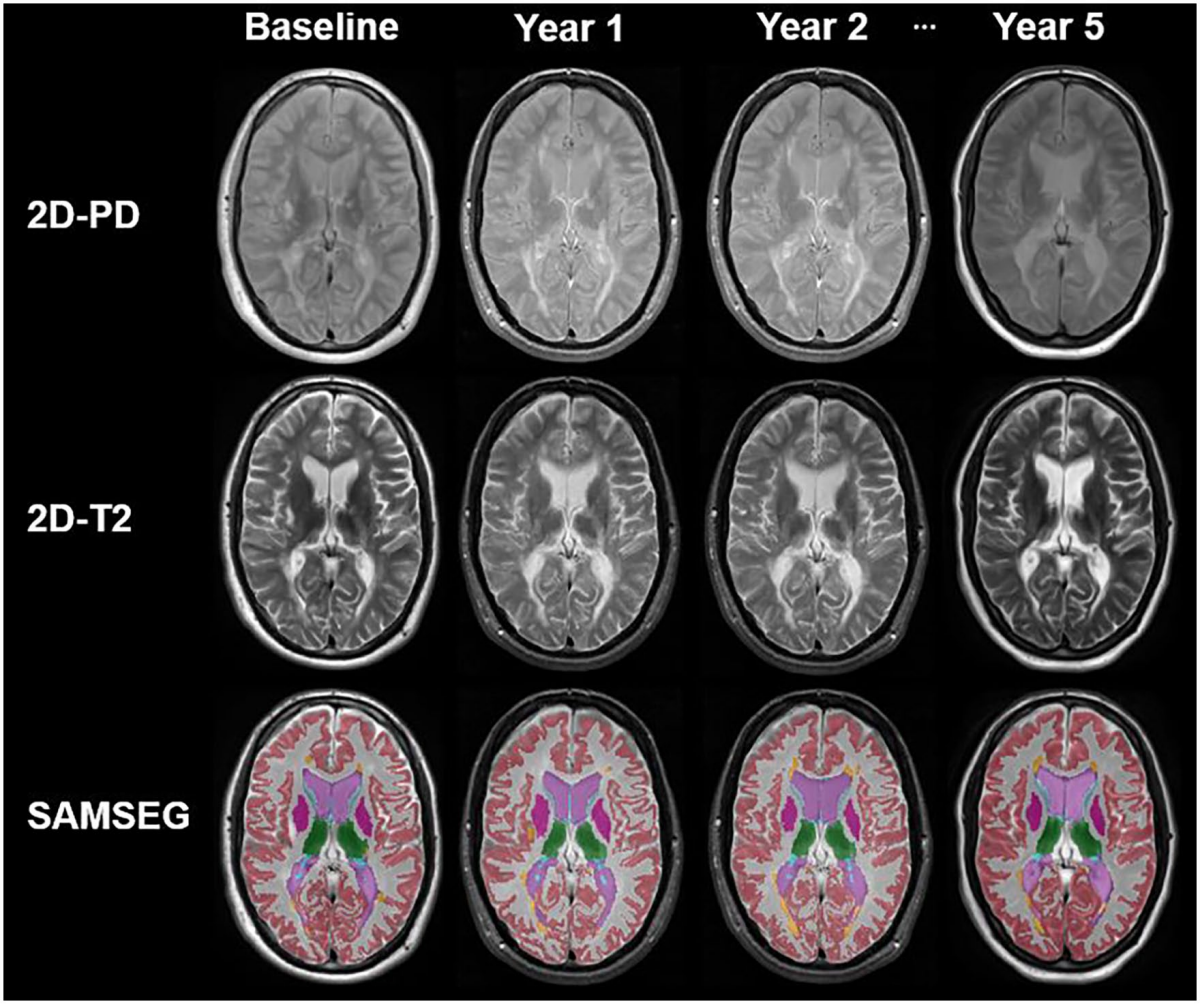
Example of longitudinal brain segmentation on 2D PD/T2-weighted images with sequence-adaptive segmentation method (SAMSEG). red: cortex; white: white matter; purple: ventricle; green: thalamus; dark purple: putamen; orange: lesions.
